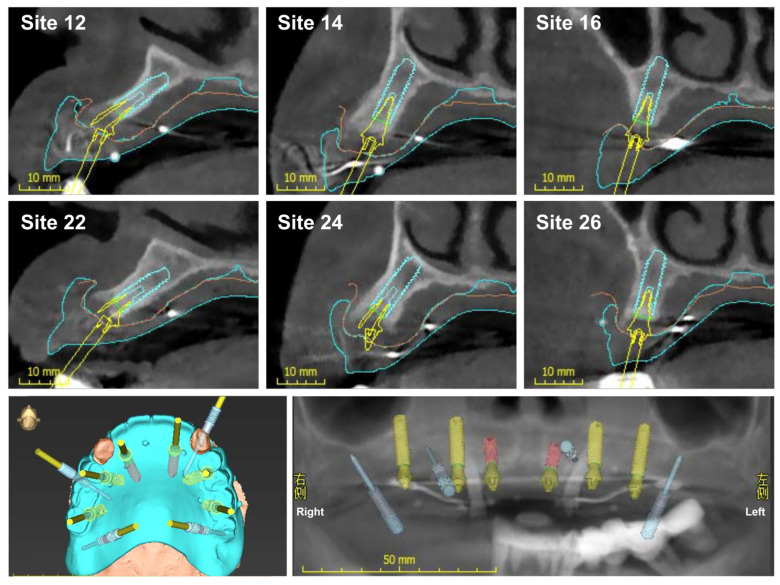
Virtual 3D planning positions for placing six implants and four metallic pins in the maxilla. In the panoramic radiograph, red implants show a diameter of 3.4 mm, yellow implants indicate a diameter of 4.0 mm, and blue ones represent metallic pins.
